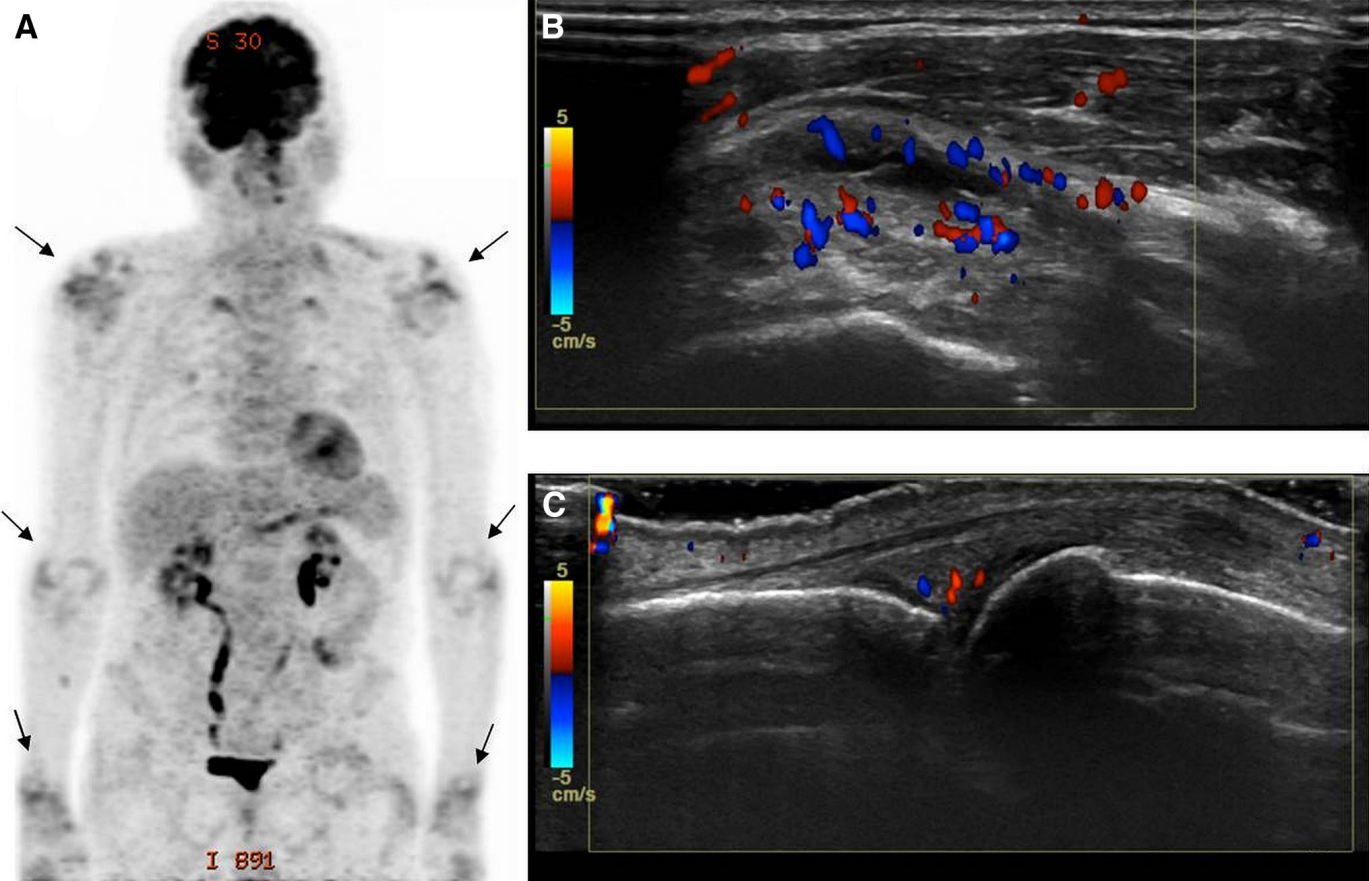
76-year-old man with arthritis after 21st dose of nivolumab. a PET-CT shows oligoarthritis with increased FDG uptake in the shoulders, elbows and wrists (*arrows*). b Shoulder arthritis with thickened synovial and increased vascularity. c Metacarpophalangeal arthritis with thickened increased vascularized synovia
